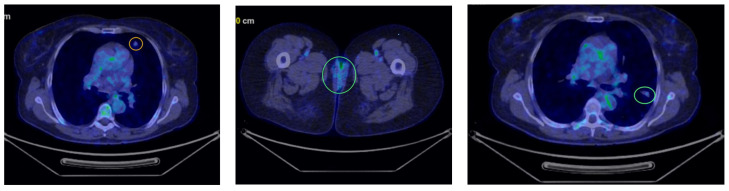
First PET-CT revealing multiple bilateral non-calcified pulmonary nodules (marked in yellow and green circles).
